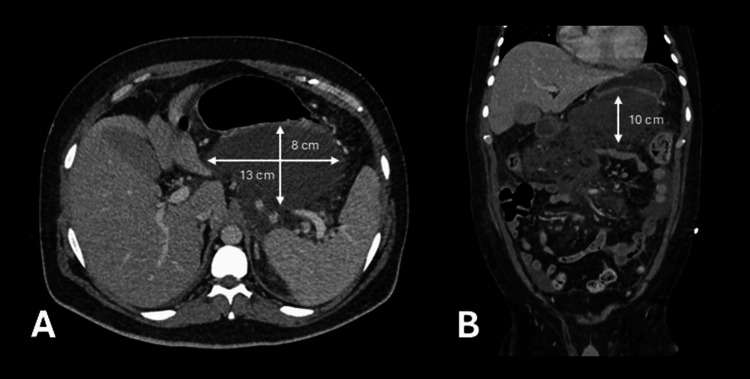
Abdominopelvic CT scan one week after symptom onset. Unfavorable progression with 50% pancreatic necrosis (CT Severity Index: 9) and a large pancreatic collection measuring 13 × 8 × 10 cm, displayed in axial (A) and coronal (B) planes.
